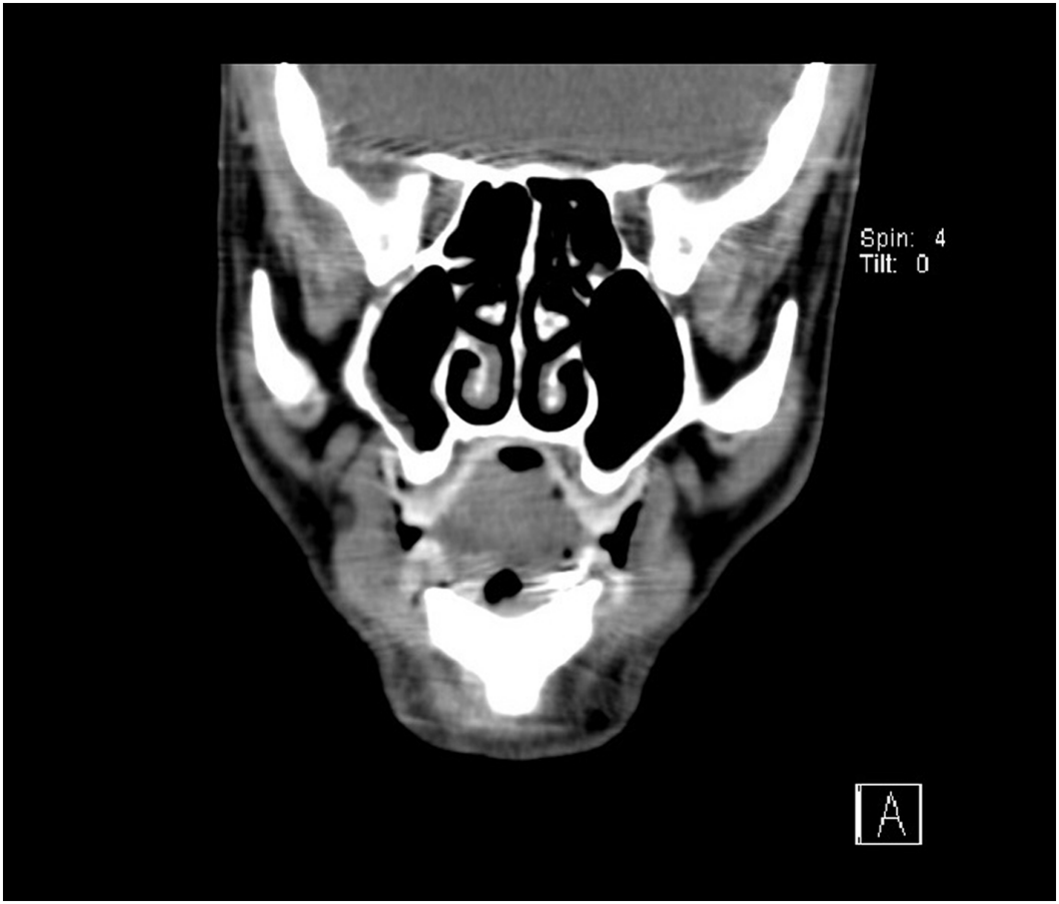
The coronal view of the paranasal sinuses' CT scan was unremarkable, without any space‐occupying lesions.
